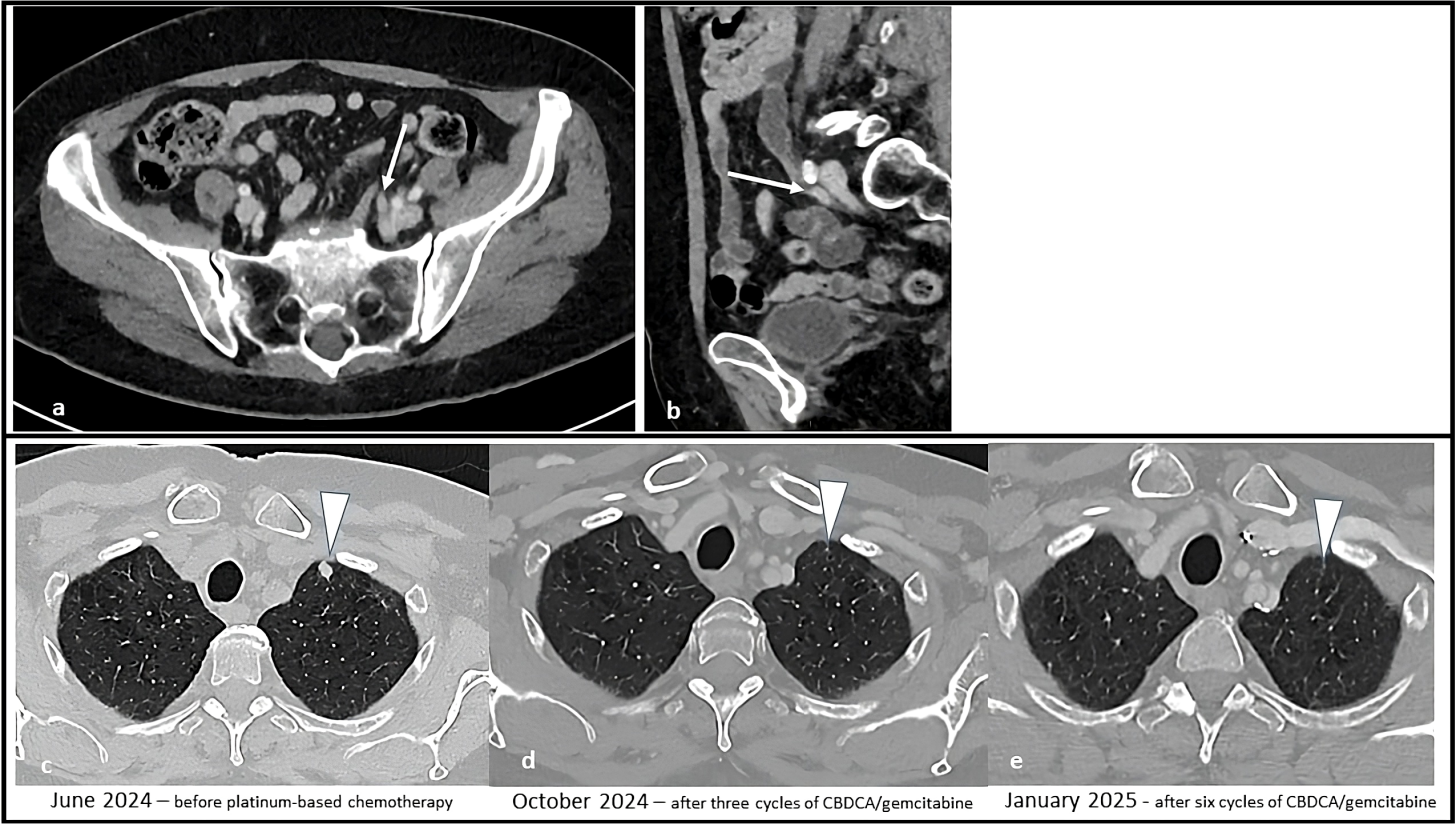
Sequential CT scans showing disease progression during treatment with enfortumab vedotin plus pembrolizumab and subsequent partial response with platinum-based chemotherapy. Solid tissue within the left ureter causing hydronephrosis (white arrow) in the axial (a) and sagittal (b) CT images. Afterwards, the appearance of solid lung nodules was noted [white arrowhead in (c)], that progressively responded to therapy (d) and disappeared (e).
